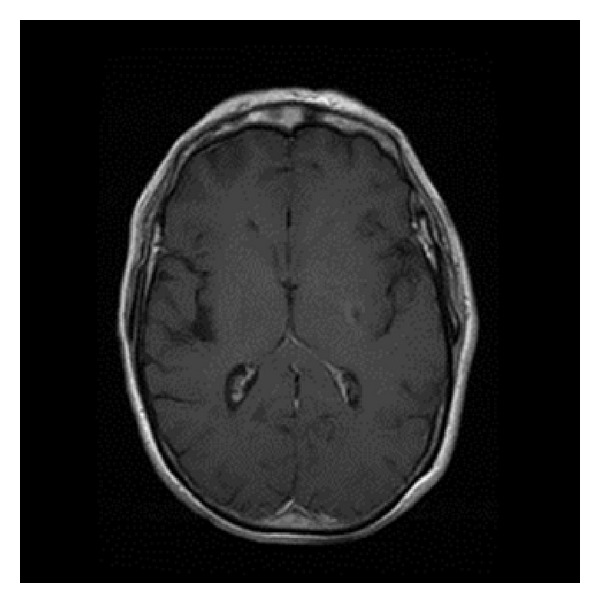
T1-weighted brain MRI showing ischaemic lesion of left posterior putamen.
